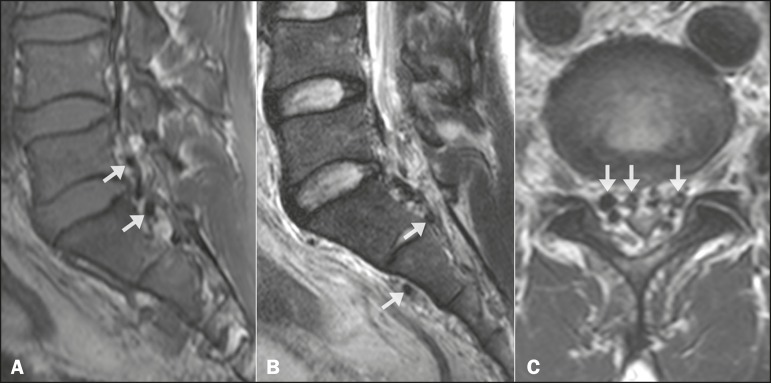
Magnetic resonance imaging, including a sagittal T1-weighted image
